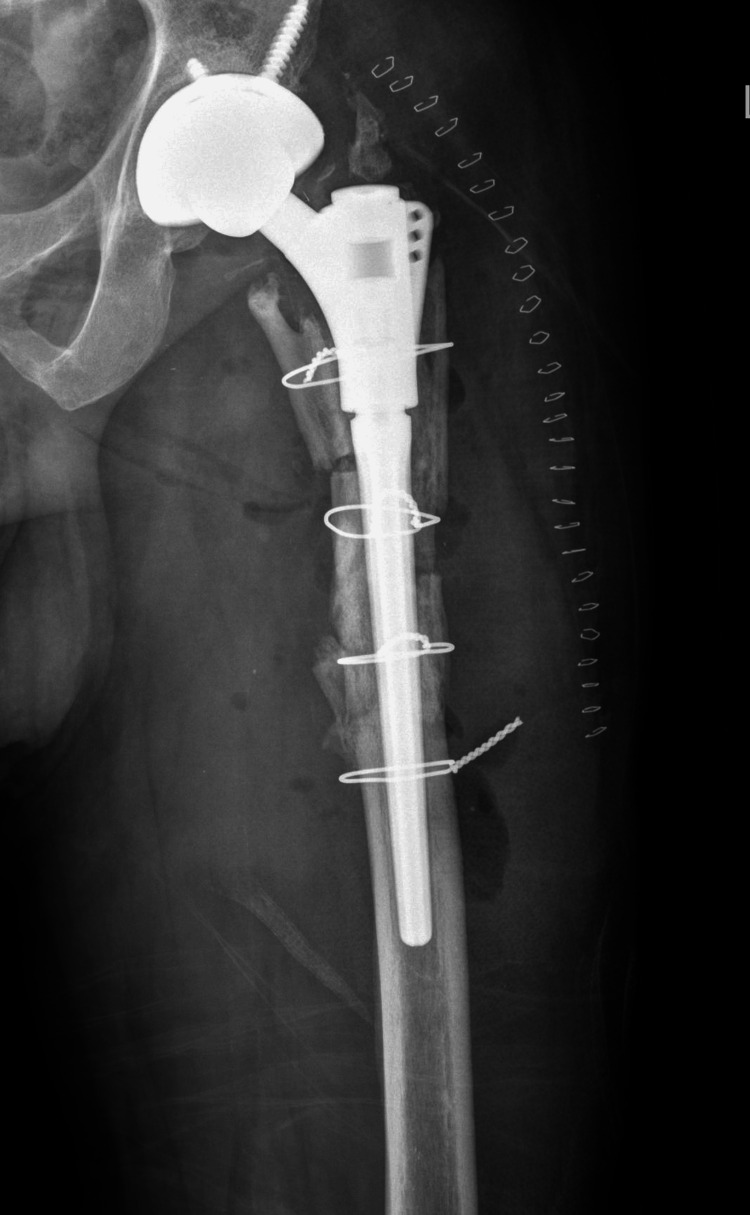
Immediate postoperative radiograph after revision total hip replacement (THR)
